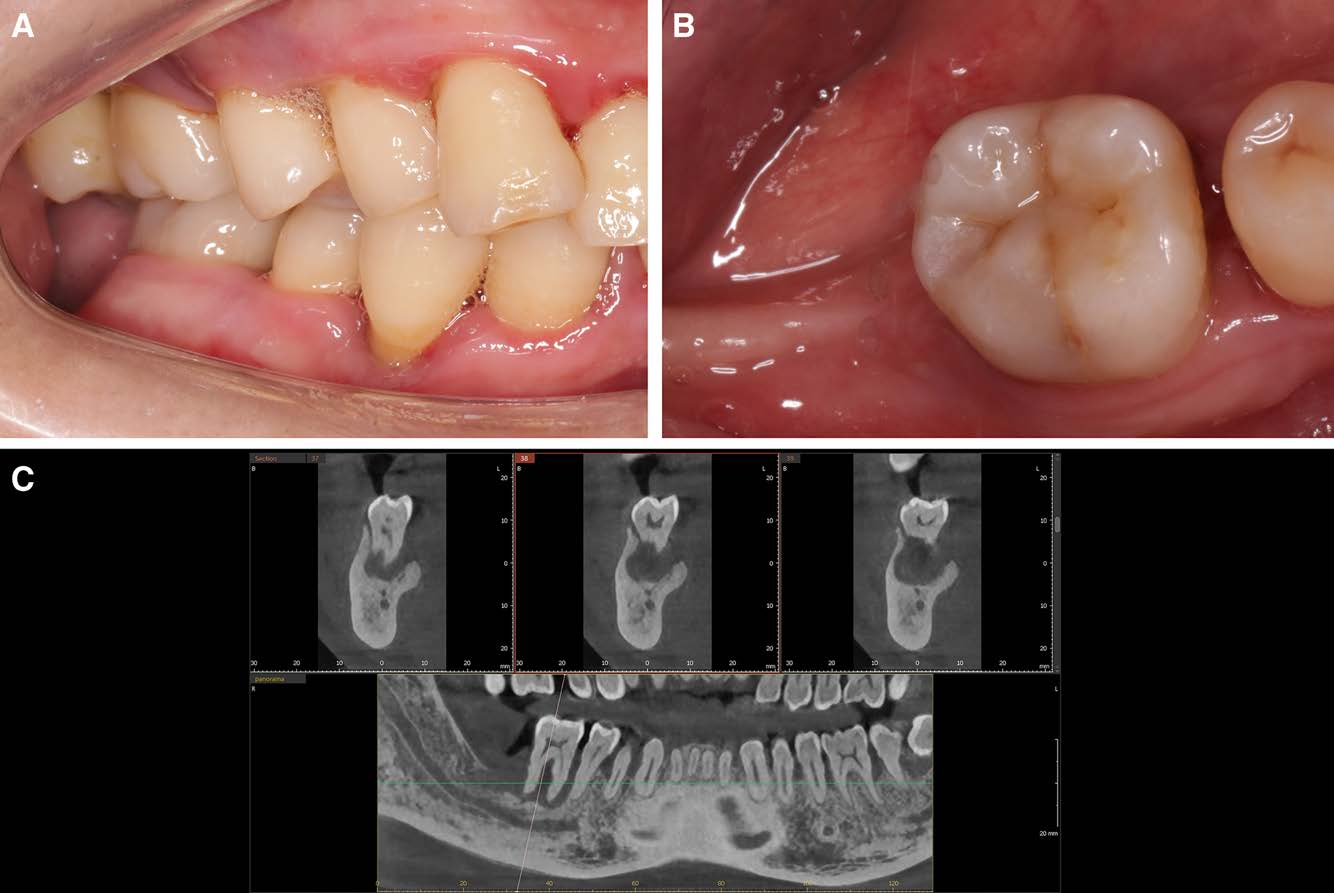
(A) View of the buccal side of the right posterior area; (B) view of the occlusal surface of tooth #46 right lower molar area; (C) preoperative assessment for tooth #46 using cone beam computed tomography (CBCT) scan.
